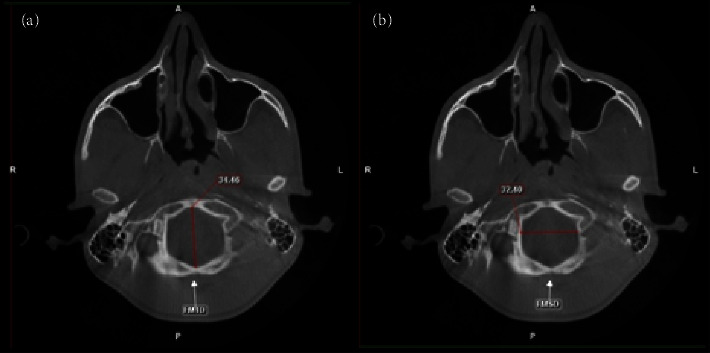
CBCT scan showing measurements of the foramen magna. (a) Foramen magnum sagittal diameter; (b) foramen magnum transverse diameter. Picture source: Department of Oral Medicine and Radiology, MCODS, Mangalore.
